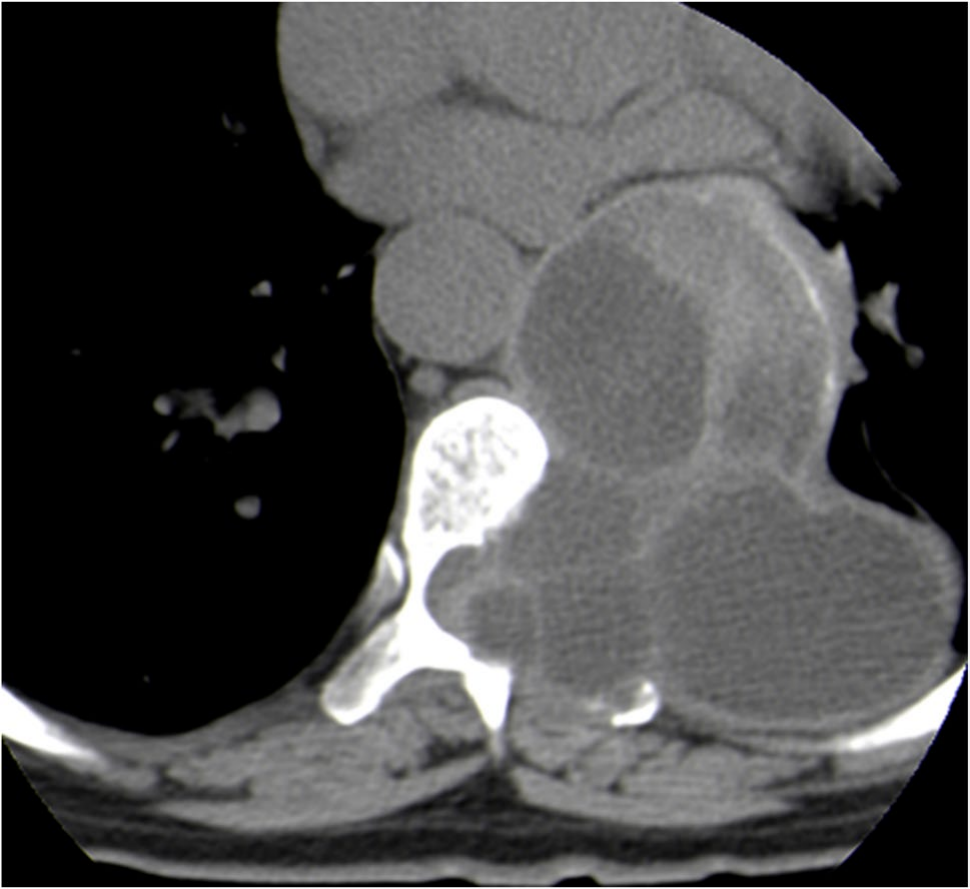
Coronal CT scan demonstrated cystic bone destruction in the first lumbar spine with a large hydatid cyst in the left soft tissue
